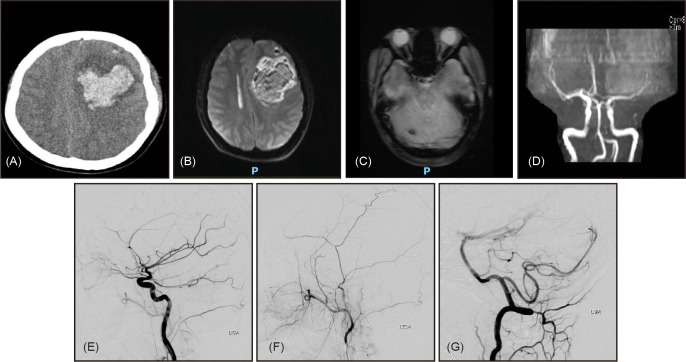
Emergency neuroimaging results of the patient. (A, B) Preoperative non-contrast (A) head CT and (B) brain MRI revealed intracerebral hemorrhage in the left cerebral hemisphere. (C) MRI also showed patchy GRE hypointensity with hemosiderin deposition in the right cerebellar hemisphere. (D) Preoperative magnetic resonance angiography revealed no evidence of aneurysm or arteriovenous malformation. (E-G) Preoperative emergency digital subtraction angiography of the left internal carotid artery (E), left external carotid artery (F), and left vertebral artery (G) similarly showed no evidence of aneurysm or arteriovenous malformation.
